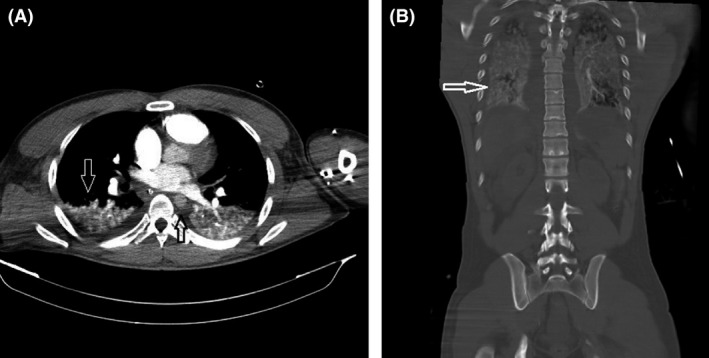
Pulmonary congestion (white arrows in A and B) and small caliber aorta (black arrow in A) are seen. Bilateral pleural effusions and contrast media filling the right side of the heart without evidence of contrast in the left side during arterial phase of the CT study are evident.
